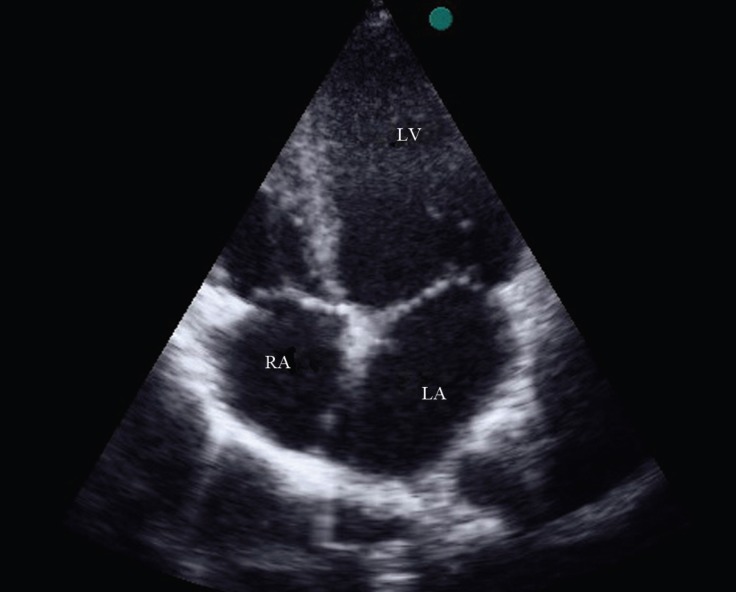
Transthoracic echocardiography in the apical 4-chamber view, showing dilated LV and LA.
